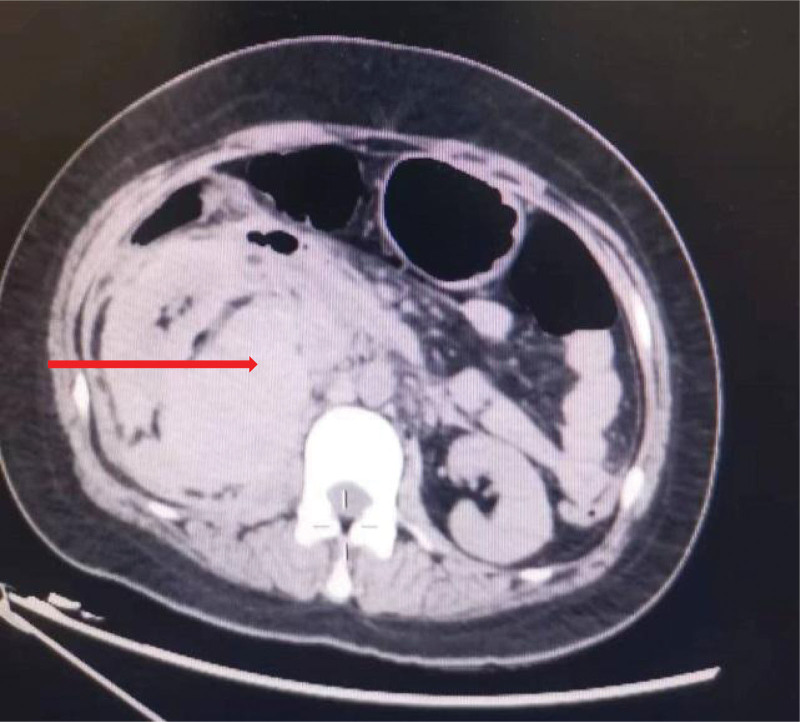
Abdominal plain scan CT shows possible rupture of the right kidney and exudation (exudation involves retroperitoneum and pelvic cavity). The arrow shows the right perirenal hematoma (size about 5.0 × 7.8 × 8.8 cm).
